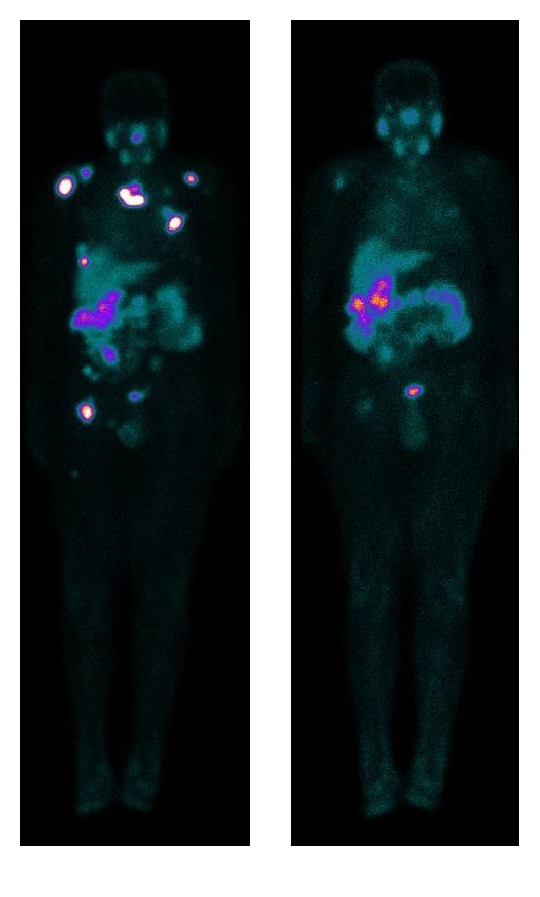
Anterior whole body ^177^Lu-PSMA scan of a 72-year-old patient with metastasised castration-resistant prostate cancer. This demonstrates normal biodistribution in the nasal region, salivary glands, liver, and spleen gastrointestinal and urinary system, with multiple PSMA-avid metastases in the prostate bed, clavicle, sternum, clavicle, vertebrae, iliac bone, and femora. An excellent response to therapy was observed after 3 cycles of ^177^Lu-PSMA-RLT with decrease in serum PSA level (from 63 to 4.25 ng/mL).
